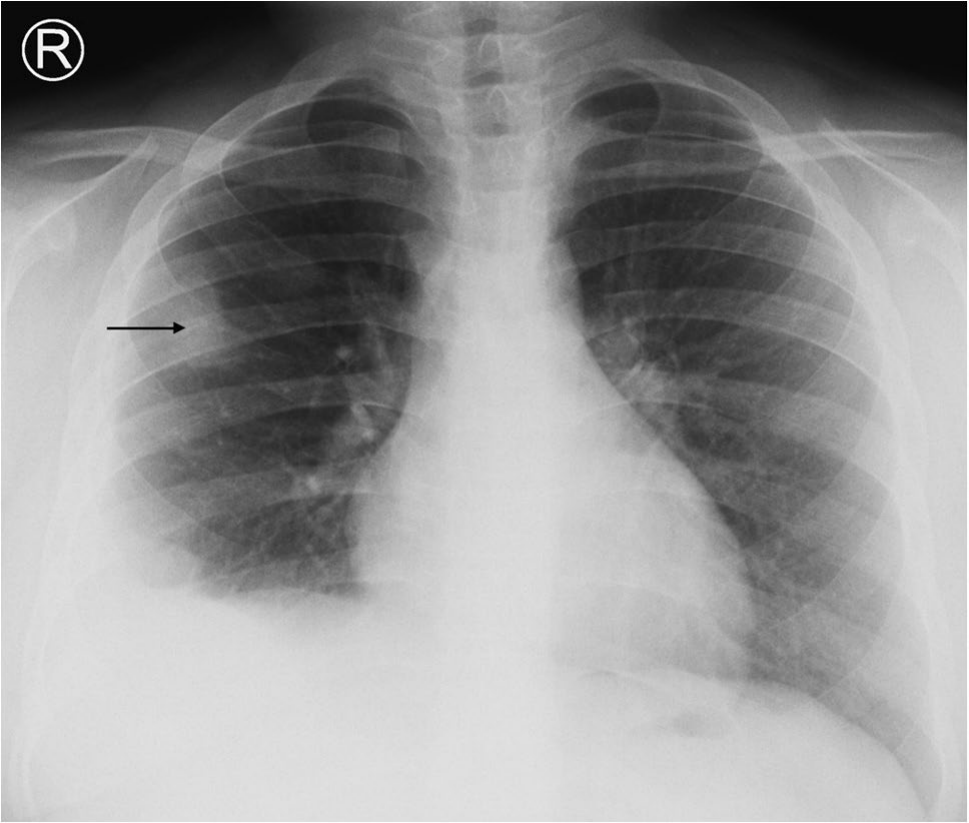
An 8-year-old boy on steroids with cough and night sweats. Posteroanterior chest radiograph shows a focal area of opacification in the right upper lobe representing a Ghon focus (*arrow*) and right pleural effusion. Elevation of the right hemidiaphragm suggests a sub-pulmonic component. Gene expert was positive for pulmonary tuberculosis
